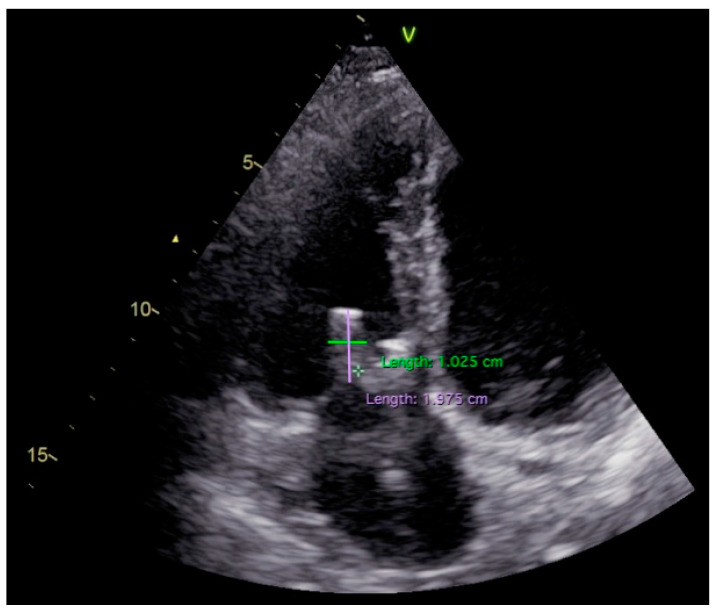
Transthoracic two-dimensional echocardiography apical four-chamber view: large hypoechogenic hyper-pedunculated mobile mass (19.5/10 mm) at the level of tricuspid valve.
